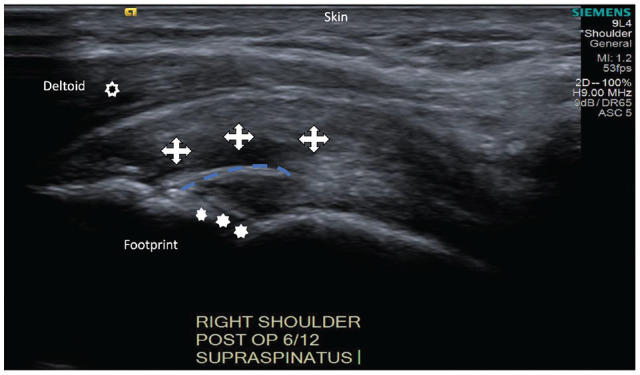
An ultrasound image showing 3 distinct supraspinatus tendon locations (crosses) along the tendon contour (blue line), humeral head (stars), and deltoid (hollow circle) measurements 3 mm apart.
